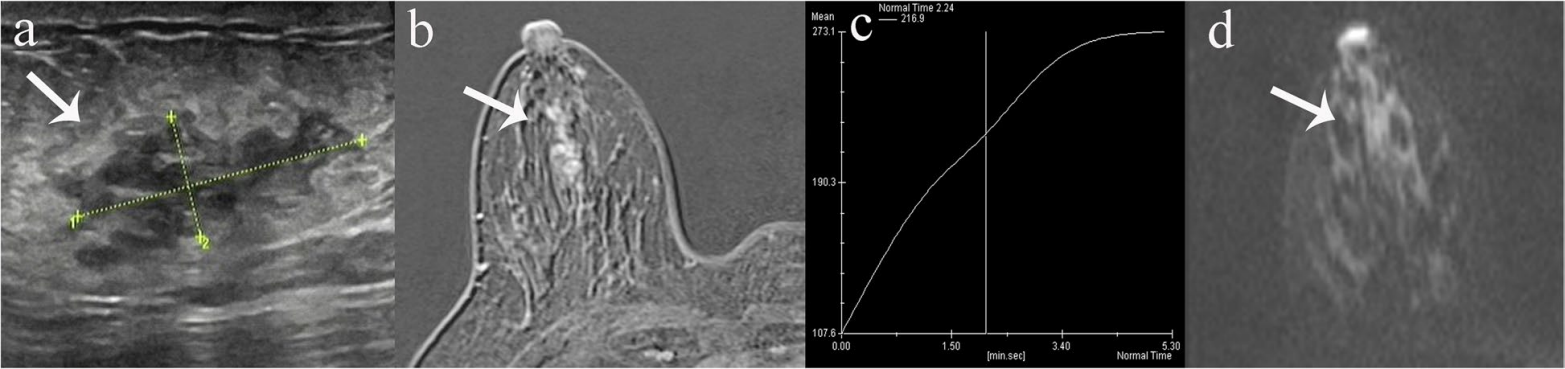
True-negative case of MRI finding for a lesion with US BI-RADS 4A category. a Gray-scale US revealed 29 mm non-mass hypoechoic area on the right breast and 4A category was given. b, c Dynamic contrast-enhanced MRI showed an irregular mass with circumscribed margin and persistent kinetics. d The lesion showed slight hyperintensity on DWI, with mean ADC values of 1.51 × 10^–3^ mm^2^/s. BI-RADS 3 category was given on MRI. Pathology revealed fibrocystic changes
